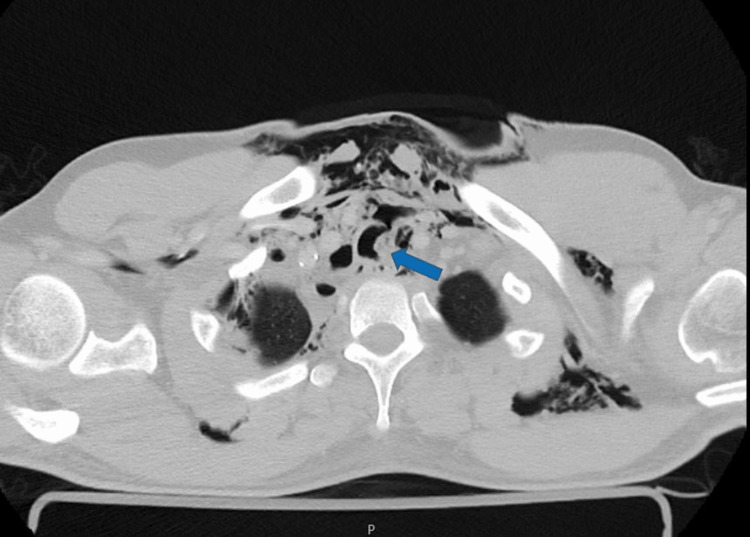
Admission thoracic CT: Tracheal laceration (axial view)
